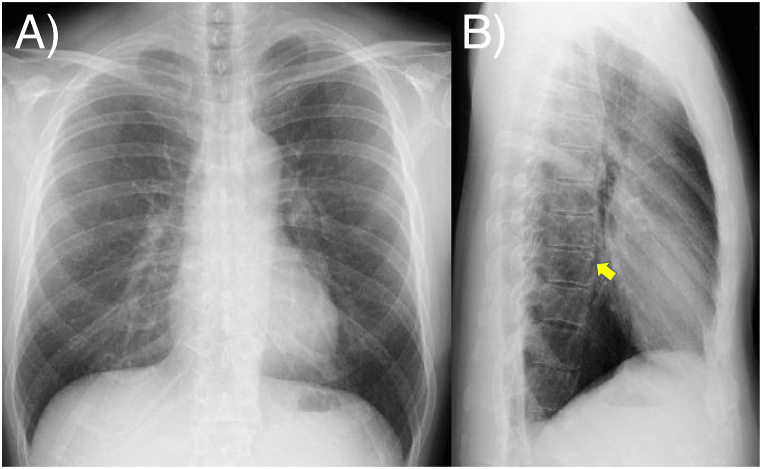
Chest radiograph A) Frontal view: no obvious nodular shadow B) Lateral view: a cavitary nodule overlapping the vertebral bodies in the lower lung field (arrow).
